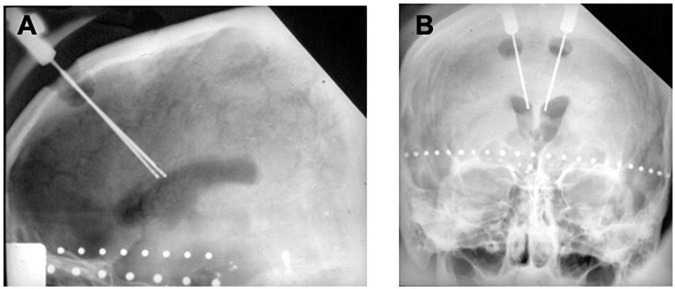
Original ballantine cingulotomy. (A) Lateral and (B) AP X-ray of electrodes in place for creation of a cingulotomy lesion.
